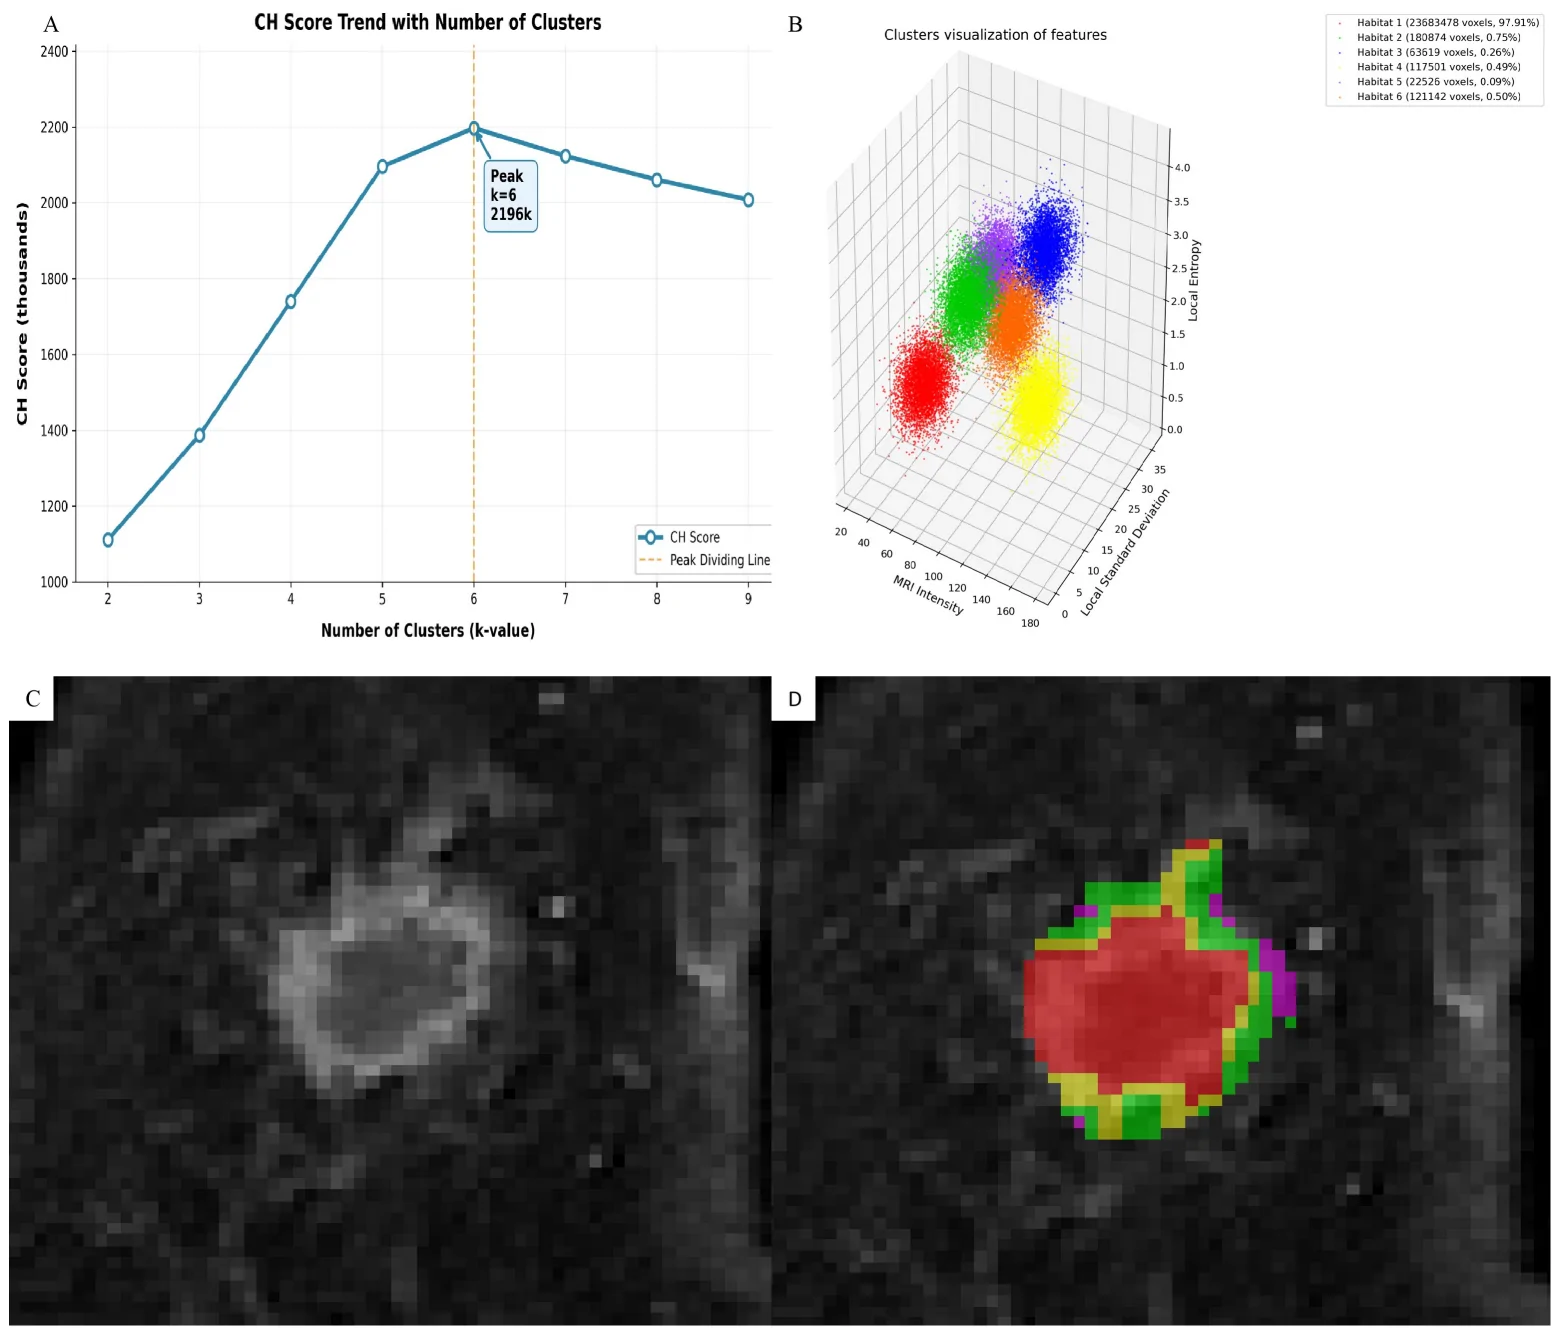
Habitat clustering results. (A) Calinski–Harabasz score line chart. (B) three-dimensional visualization of Clusters. (C) Dynamic contrast-enhanced magnetic resonance imaging. (D) Habitat imaging.
